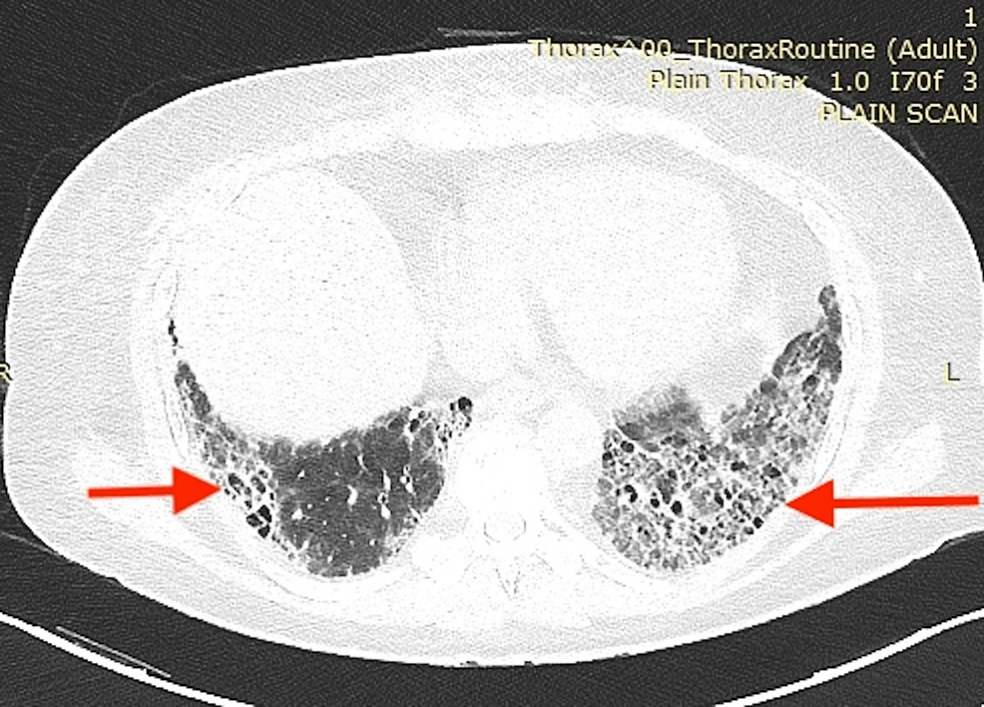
HRCT thorax showing heterogenous subpleural and basal predominant honeycombing with traction bronchiectasis in the bilateral lung HRCT: High-resolution computed tomography.
